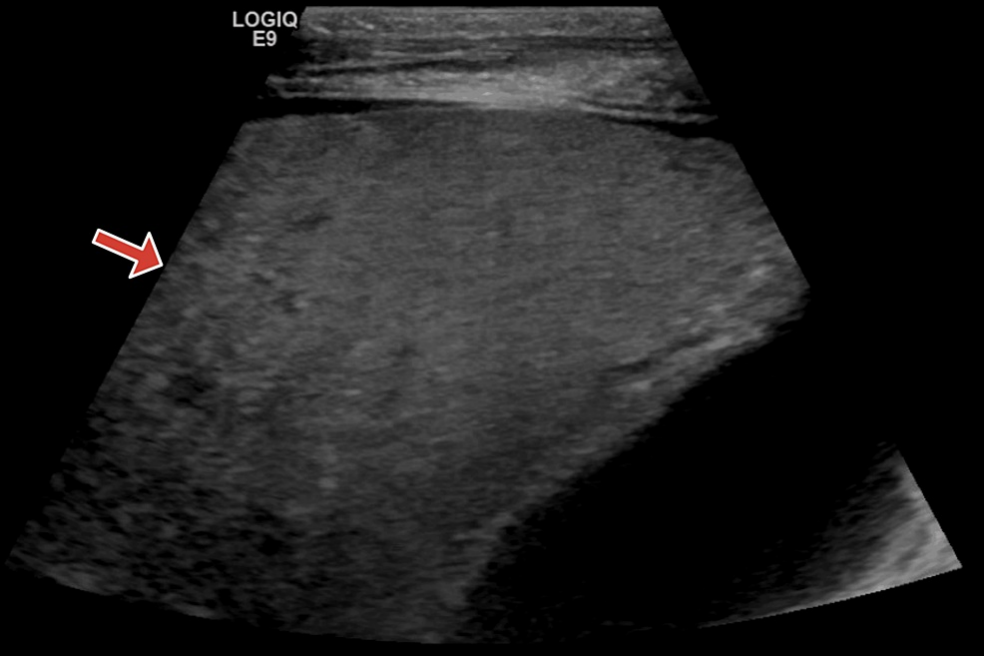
Liver ultrasound Red arrow: Mild hepatomegaly with diffuse hepatic steatosis without evidence of cirrhosis
